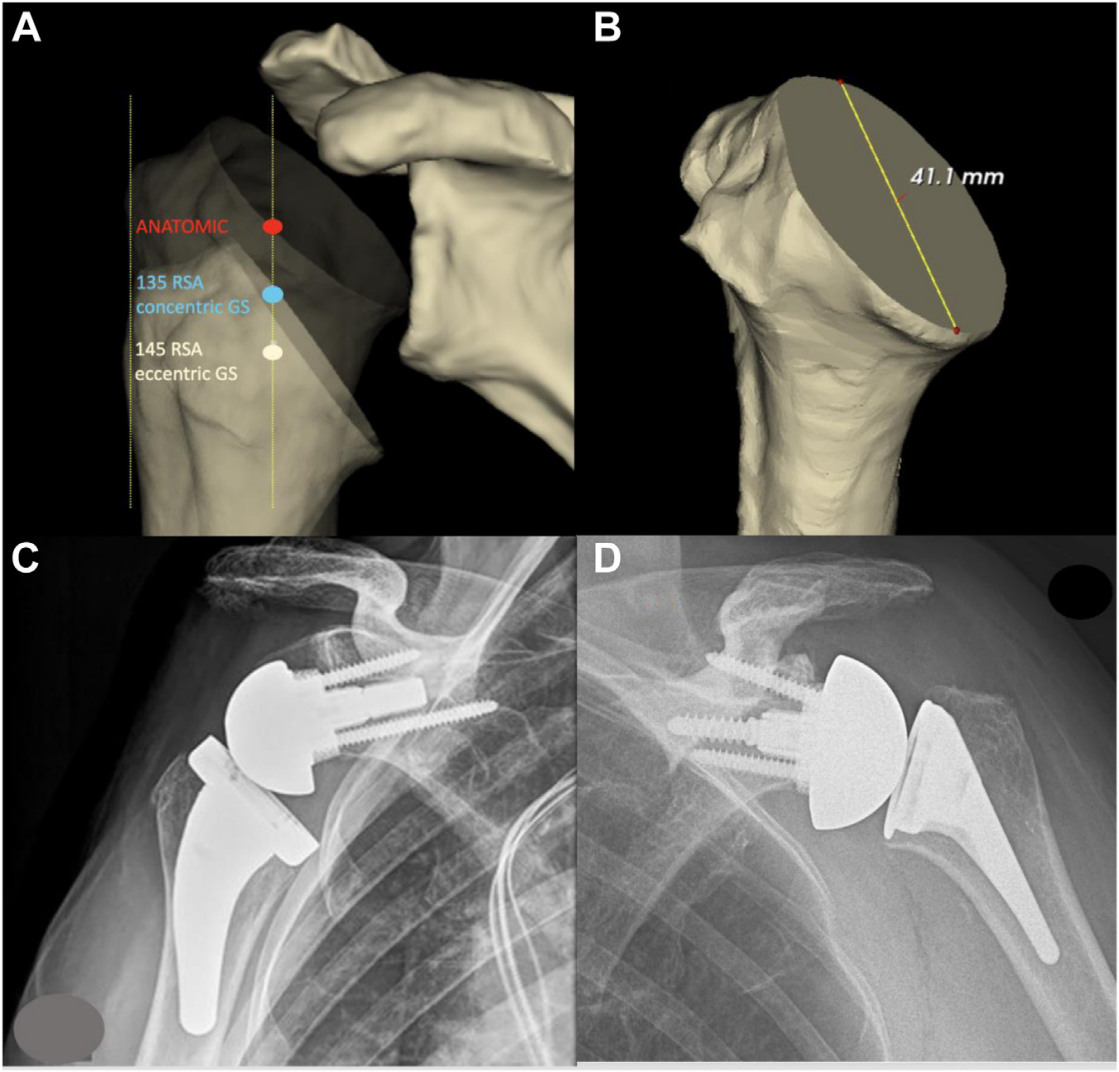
(A) Anatomic lateralization and differences of distalization in 145º (*white*), 135º (*blue*) models, and anatomic state (*red*). (B) Humeral head osteotomy diameter representing head size. (C-D) Example radiographs of 145º and 135º RSA corresponding to 145º (*white*) and 135º (*blue*) models in (A). *RSA*, reverse shoulder arthroplasty.
